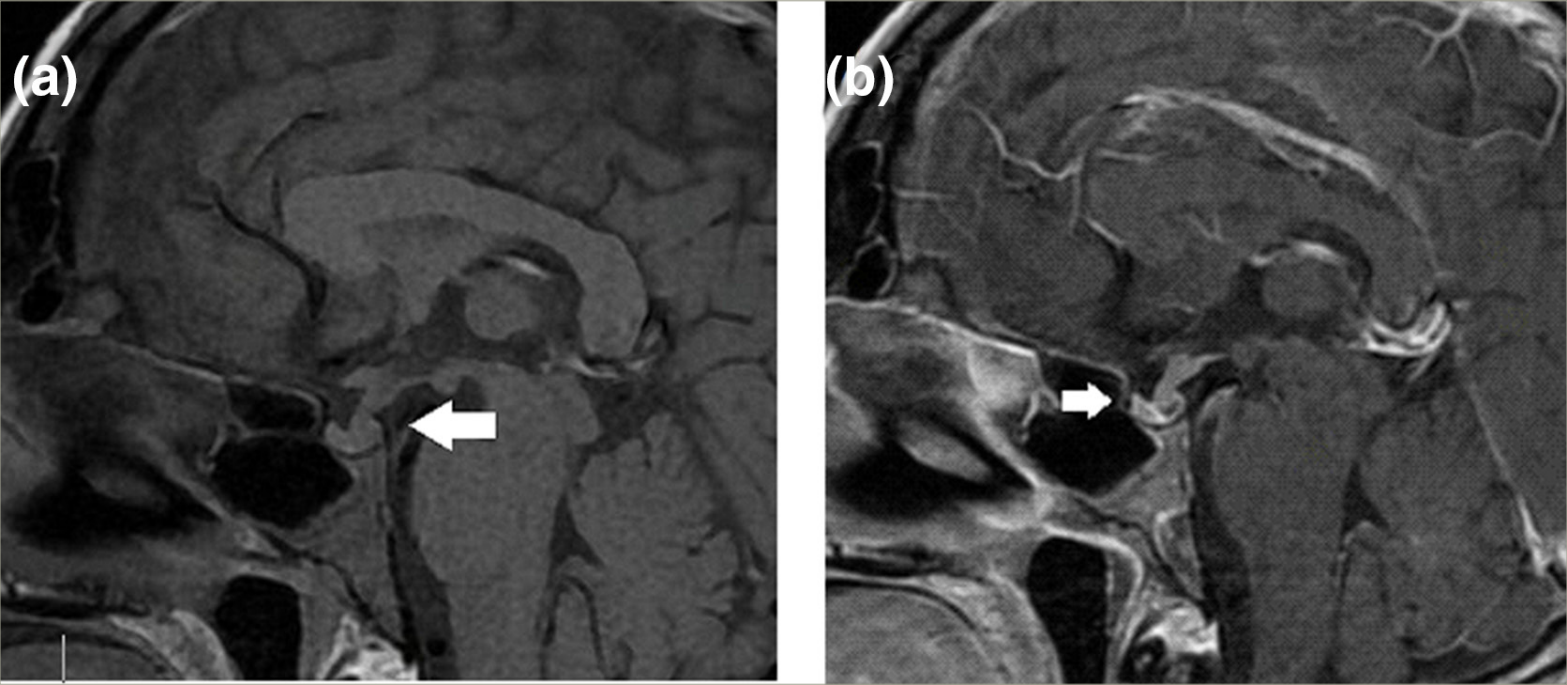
Pituitary magnetic resonance imaging
